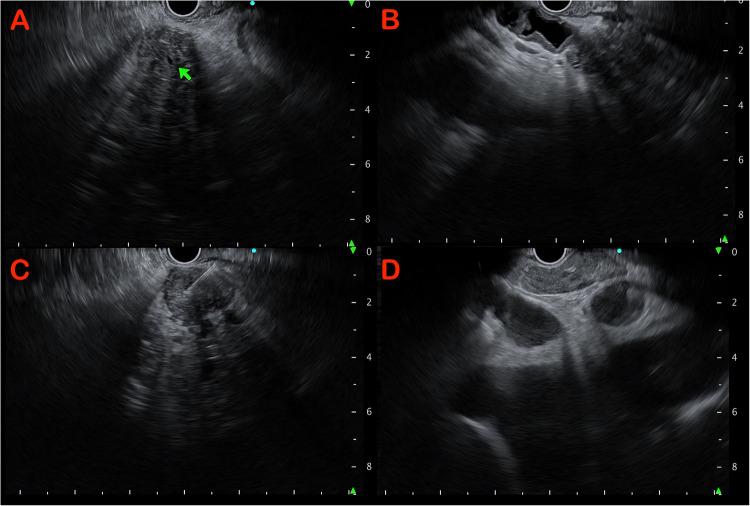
(A) Pancreatic head showing heterogenous echogenicity with undilated pancreatic duct. (B) Diffuse thickening of the bile duct seen on endoscopic ultrasound. (C) Biopsy taken from the pancreatic body which also appears heterogeneously hypoechoic. (D) Enlarged sub-carinal lymph node which was reactive seen in association with autoimmune pancreatitis
